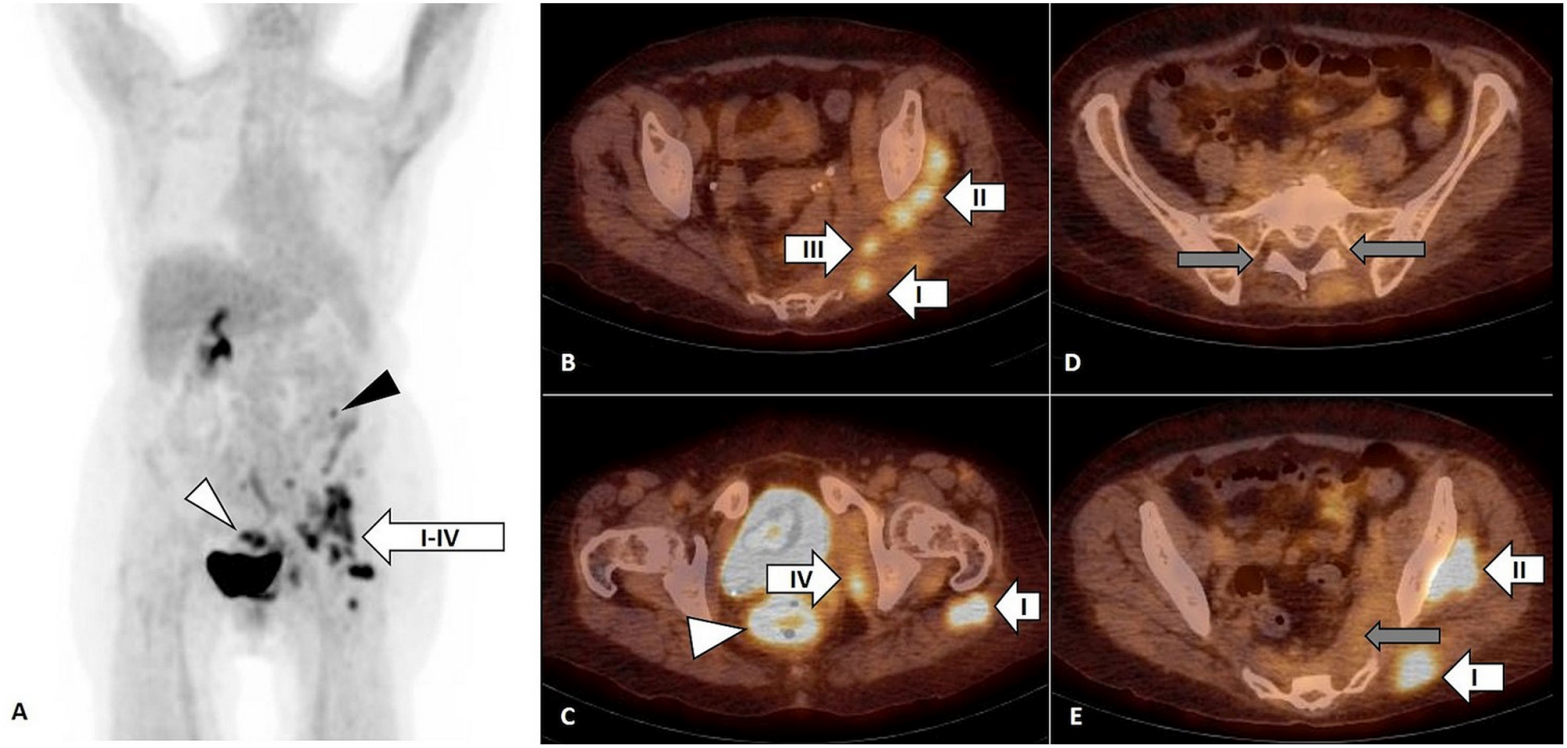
FDG PET, maximum intensity projection (MIP) (A) and FDG PET/CT, axial slice (B–E). Focal FDG uptake by infiltrates in left gluteus maximus (I), medius (II), piriformis (III), and internal obturator (IV) muscles [(A–C,E), arrow] confirmed by biopsy as diffuse large B-cell lymphoma (DLBCL). The FDG uptake in descending colon [(A), black arrowhead] had no correlate on subsequent colonoscopy and the FDG uptake in rectum [(A,C), white arrowhead] was related to pseudomembranous colitis as a complication of previous antibiotic treatment. The FDG uptake by lumbosacral plexus observed on previous FDG PET/CT was not present anymore [(D,E), gray arrow].
